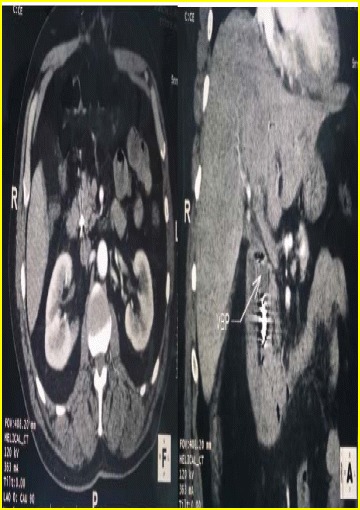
Axial (*Left picture*) and Frontal (*right picture*) CT-scan images, showing a hyper-dense obstacle in the bile duct
